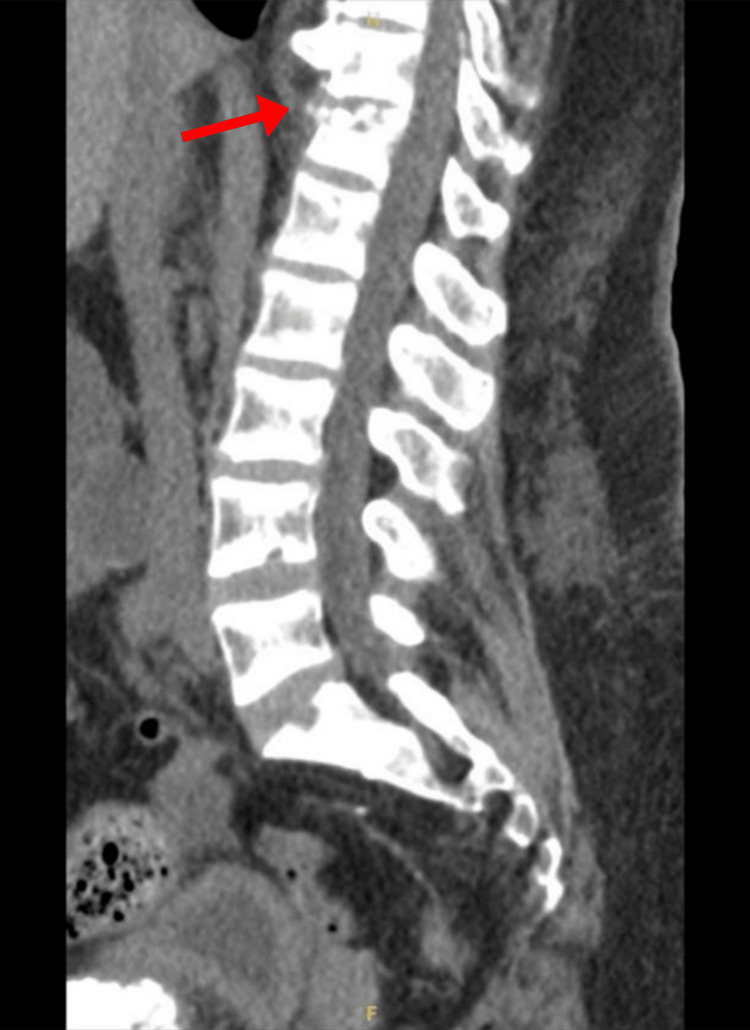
CT of the lumbar spine demonstrates T12 superior endplate compression fracture and “rugger jersey spine” appearance due to vertebral bone resorption from chronic hyperparathyroidism.
